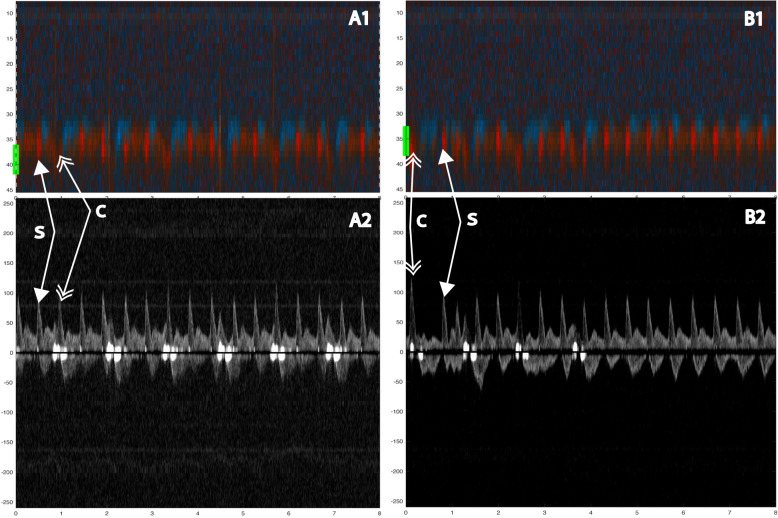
Color m-mode showing spontaneous circulation during mechanical compression. Two eight-second examples. Color M-mode (A, C) with the corresponding Doppler specter from the chosen depth below. Velocities are given in cm/s. In B and D, a narrow-dashed line indicates chest compressions and a wide-dashed line indicates a circulatory check (B, D). The two arrows: the single-tip arrow indicates spontaneous circulation, and the double-tip arrow indicates chest compression. M-mode (A) from 8-s mechanical 100 compressions with corresponding Doppler specter (B). After 4 s, the transition from compressions to circulatory check (C, D)
